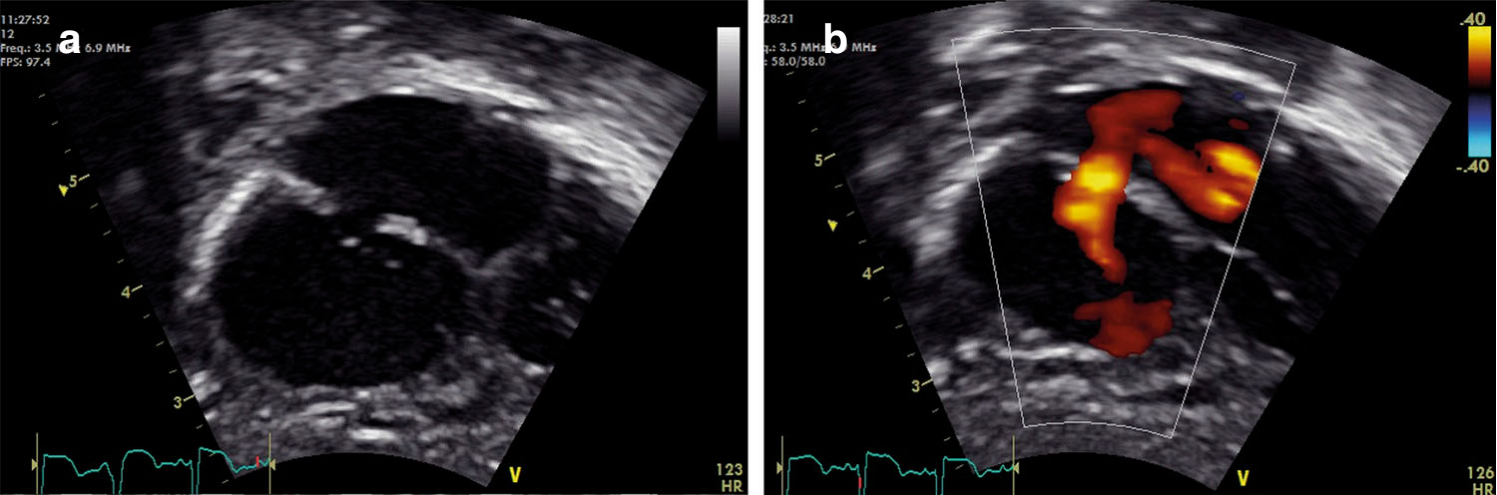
Subcostal atrial view 2D (a) and color Doppler showing left-to-right interatrial shunting (b)
